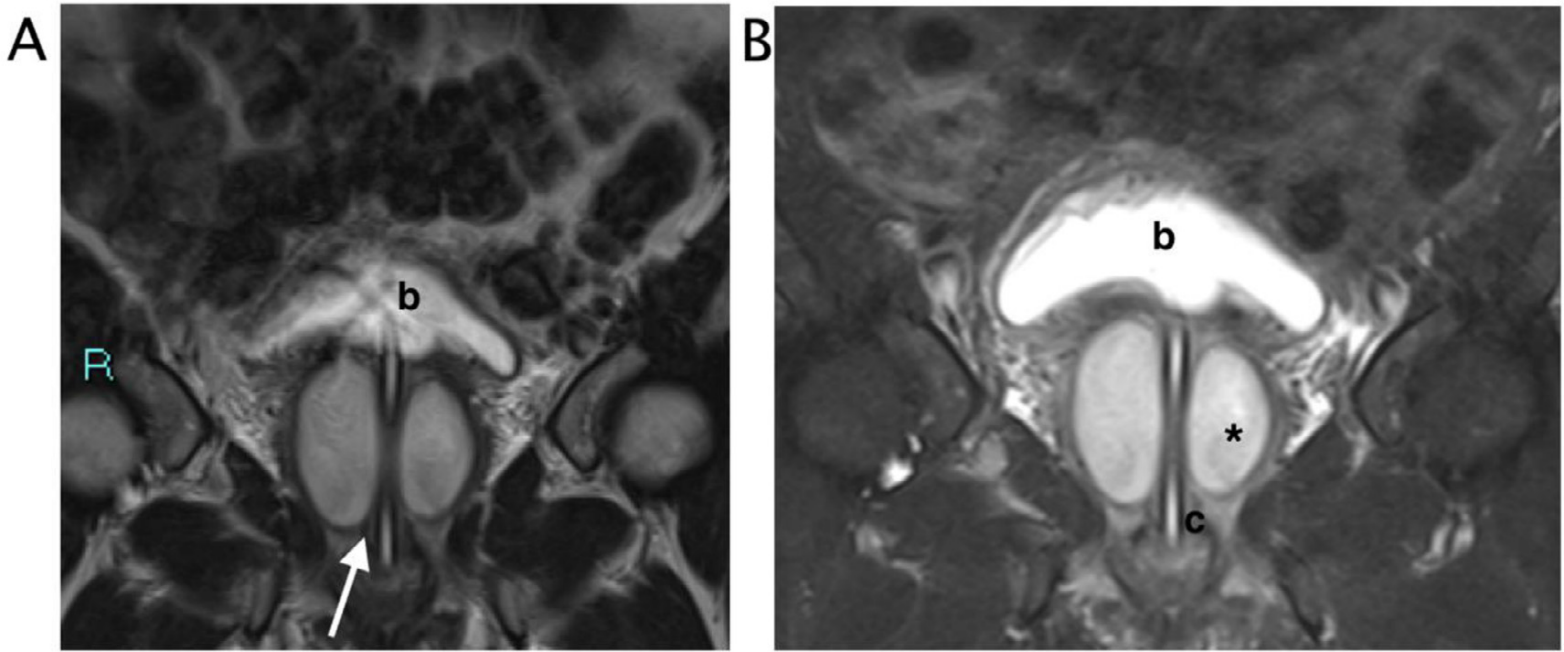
MRI. The bilocular fluid collection is located just laterally to the external urethral meatus and inferior to the pubic symphysis. (A) Coronal T2 Turbo-spin-echo sequence with selective fat suppression. (B) Coronal T2 Turbo-spin-echo sequence. The fluid filled collection with internal mildly heterogenous hyperintense signal (black asterix) is localized laterally to the urethra (white arrow), the catheter line (c) run in the middle. Bladder (b).
